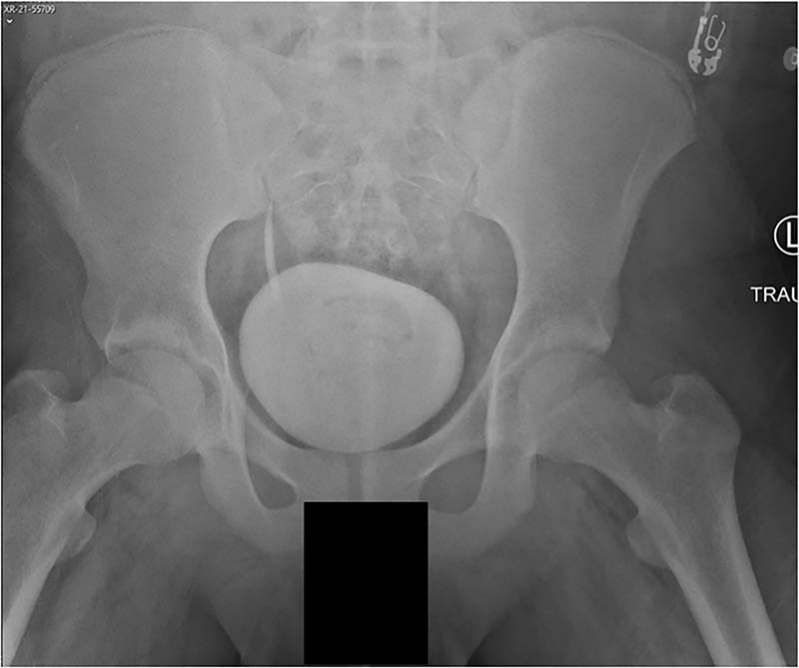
AP pelvis radiograph, modified Oxford Grade 25.
